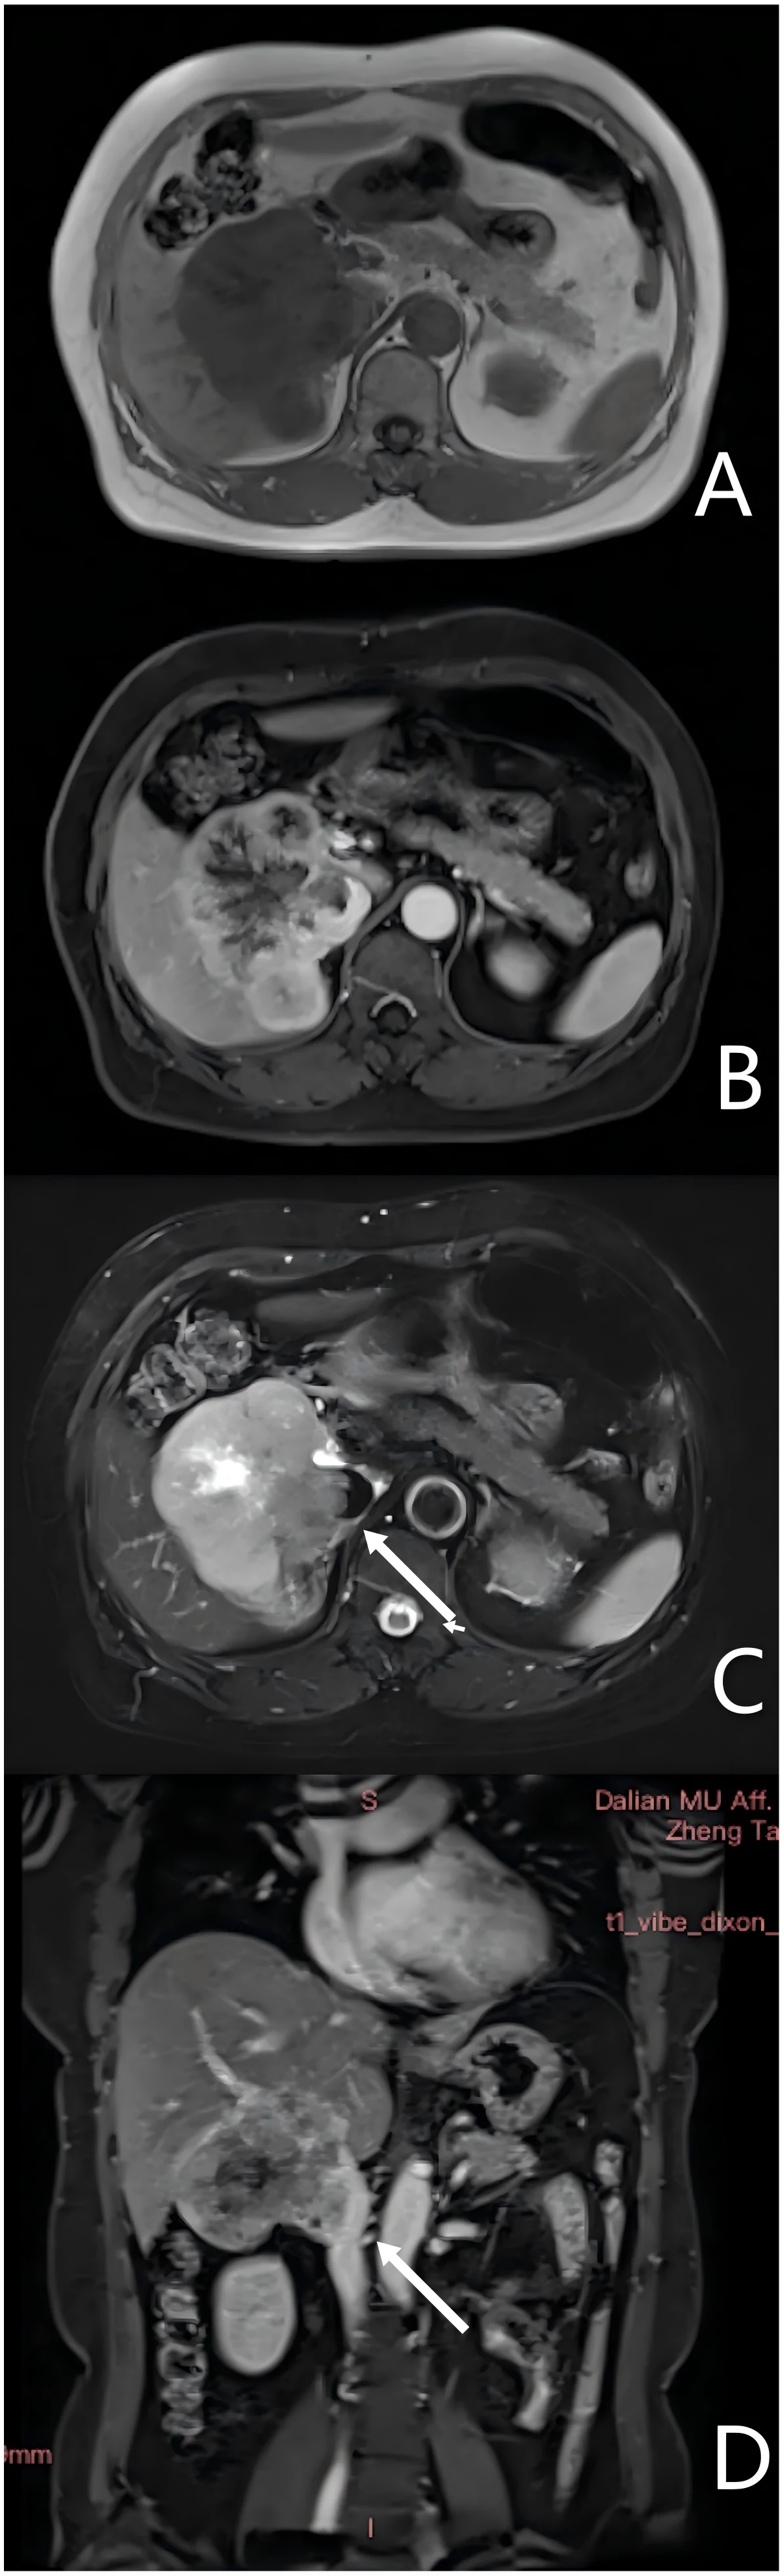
Magnetic resonance imaging (MRI) findings. (A) Axial T1-weighted image shows a hypointense mass in the right hepatic region. (B) Axial contrast-enhanced T1-weighted image demonstrates heterogeneous enhancement. (C) Axial T2-weighted image reveals heterogeneous hyperintensity within the mass, with evidence of tumor involvement within the IVC lumen (arrow). (D) Coronal contrast-enhanced T1-weighted multiplanar reconstruction (MPR) shows tumor continuity with the IVC lumen (arrow), indicating IVC origin.
